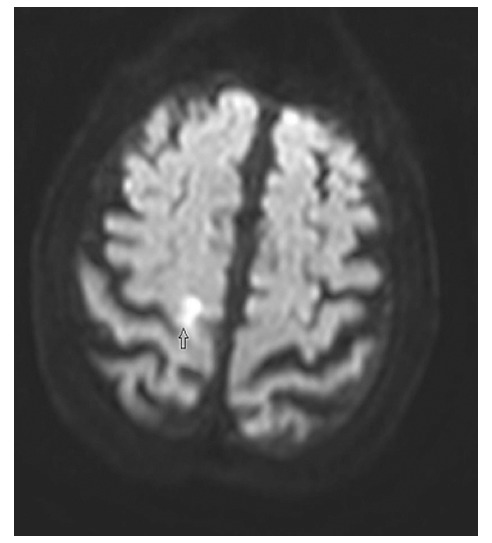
Diffusion-weighted magnetic resonance imaging of the patient revealed a focal high intensity signal in the right precentral gyrus at the high convexity area of the brain cortex.
